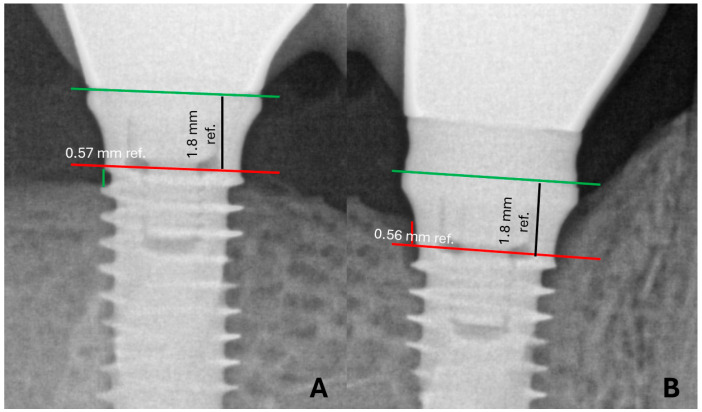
Methods for measuring bone height in an intraoral X-ray image of a lower implant. (A) Measurement below the implant platform and (B) above.
